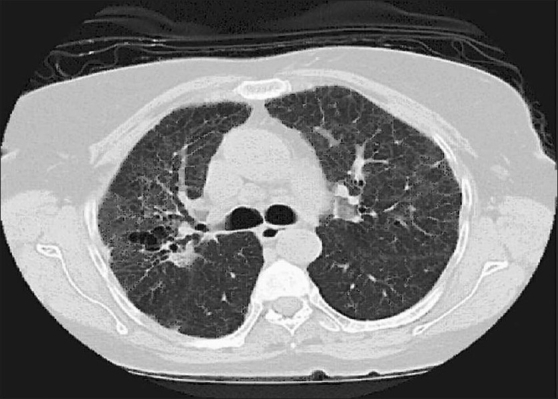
Patient 3. HRCT scan obtained at the level of tracheal bifurcation demonstrating fibronodular pattern with traction bronchiectasis. Mediastinal lymph nodes enlargement are seen. Clinical characteristics of the patient are shown in Table 4
